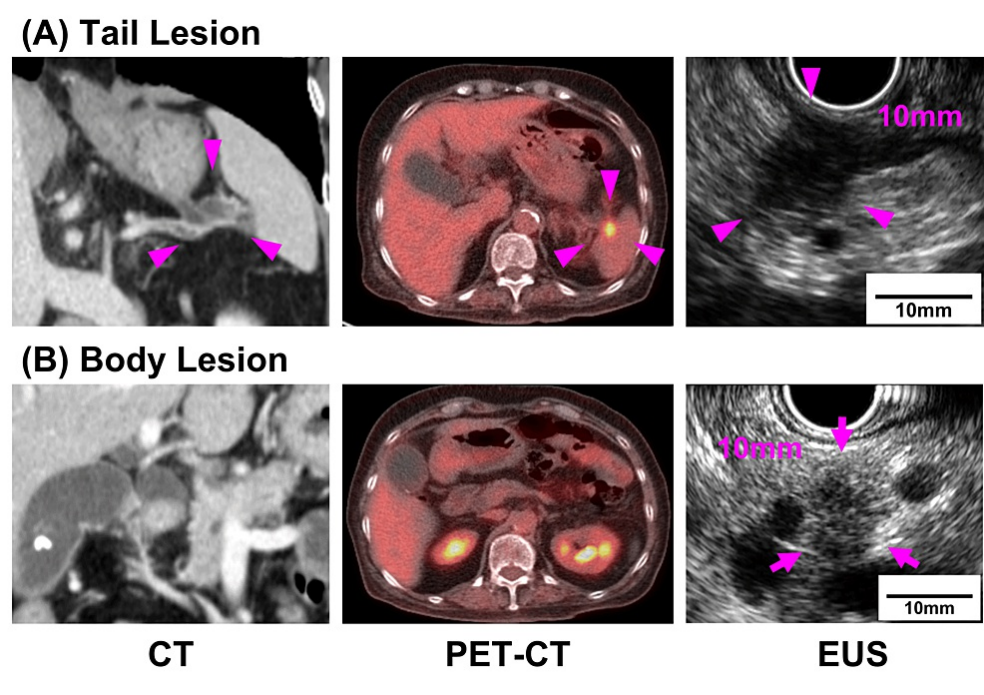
Two lesions in the pancreatic tail and body on CT, PET-CT, and rechecked EUS imaging. The lesion of the pancreatic tail (arrowhead) was detected by all imaging (A). However, the lesion of the pancreatic body (arrow) was detected only by EUS (B). CT: computed tomography; EUS: endoscopic ultrasound; PET: positron emission tomography
